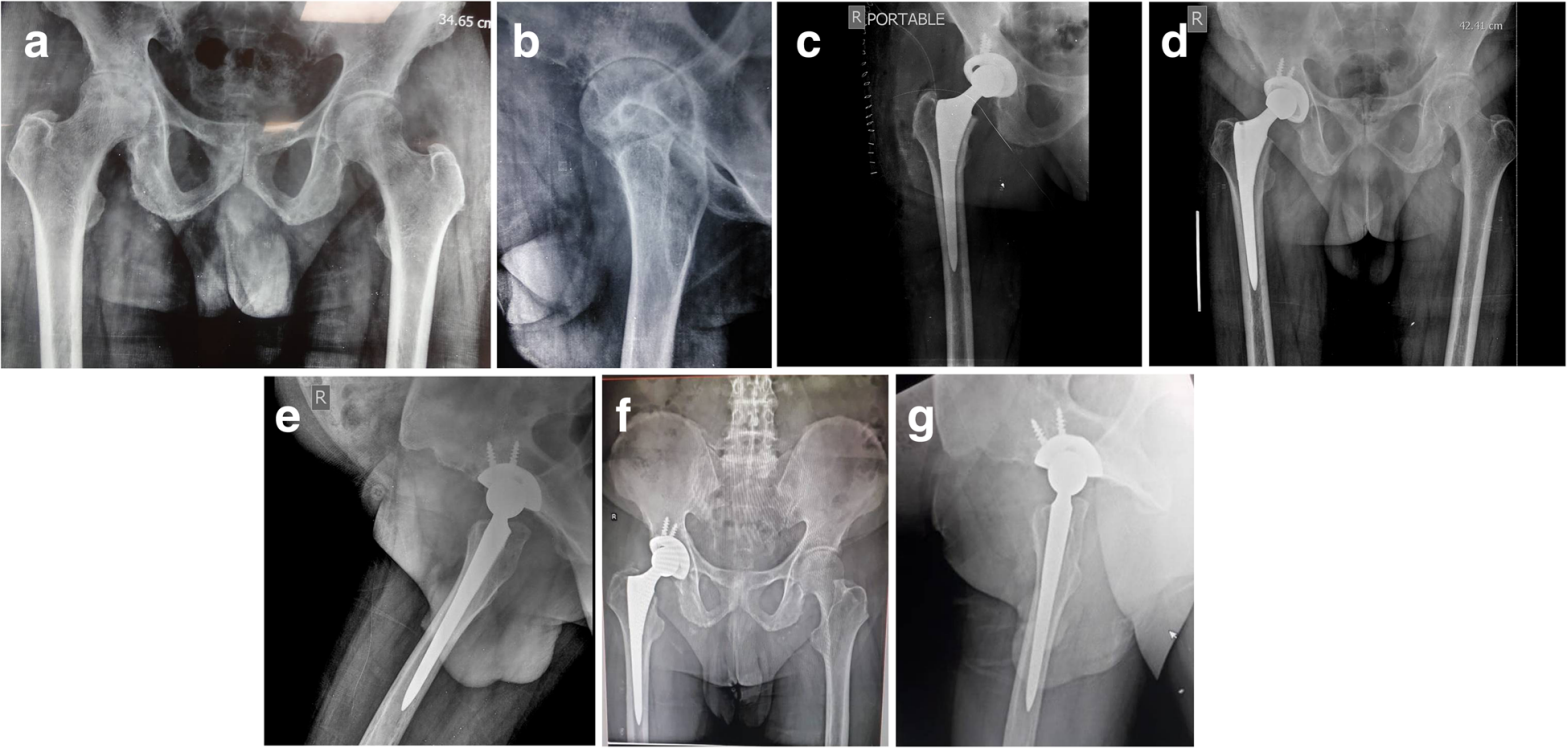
Pelvis and both hips on X-rays. a preoperative anteroposterior view; b lateral view c anteroposterior view immediately after surgery; d anteroposterior view 5 years after surgery; e Lateral view 5 years after surgery; f anteroposterior view 10.5 years after surgery; g Lateral view 10.5 years after surgery
